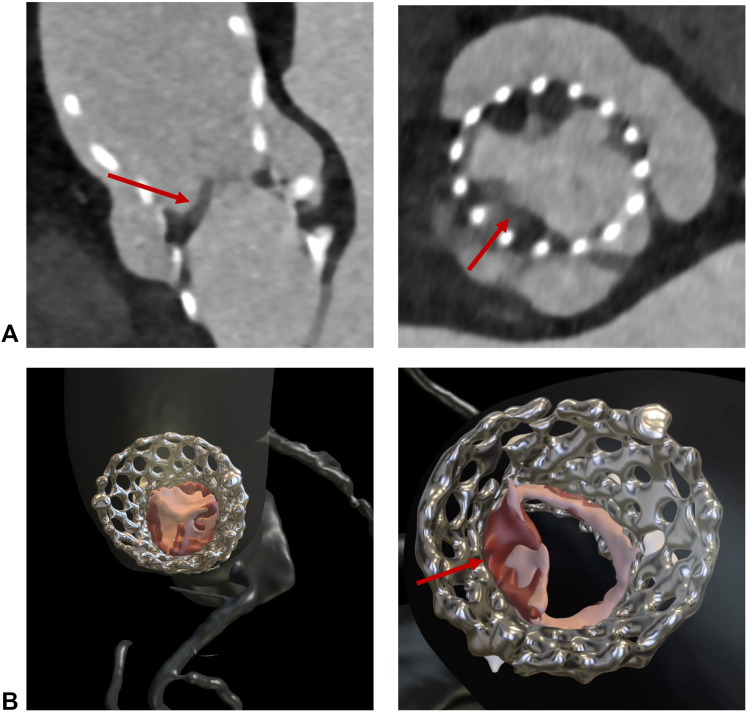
A case of subclinical thrombosis determining moderate RELM visualized with (A) MDCT and (B) multiparametric digital approach. *MDCT,* Multidetector computed tomography.
